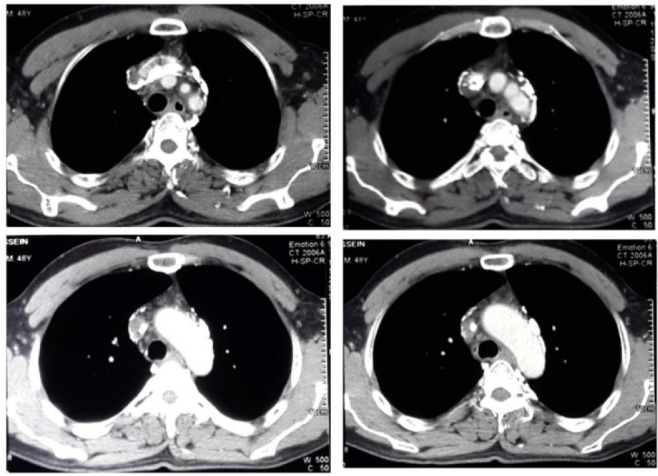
Chest CT scan with IV contrast shows irregular filling defect and thrombosis in the subclavian vein and superior vena cava
